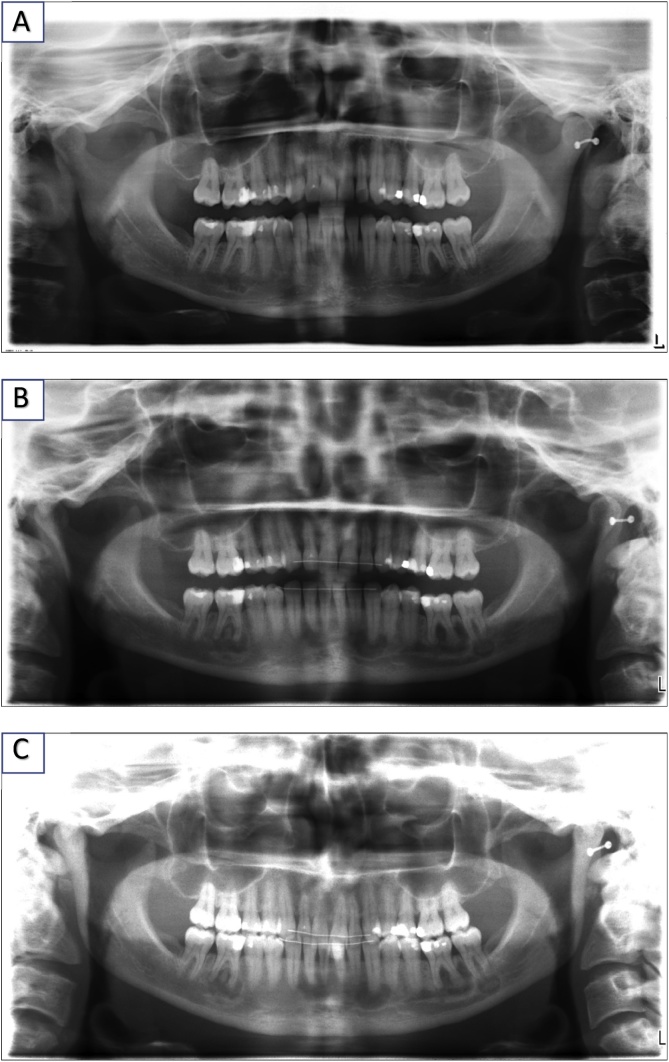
A: Panoramic radiograph acquired over 12 years before surgery. Note the elucidations at the first molars in the mandible. B. Panoramic radiograph one year before surgery. Note the increasing of the FCOD in region 36/46; 31; 42. C. Panoramic radiograph shortly before the operation.
